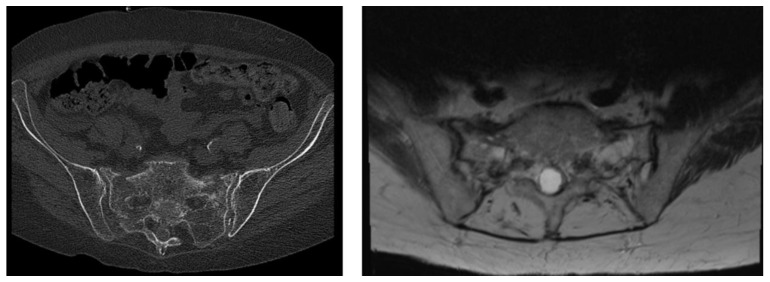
An 82-year-old patient presenting with a fragility fractures of the pelvis (FFP) type IV or OF4 (OF-Pelvis classification) with displaced bilateral posterior lesions. The bilateral fractures are seen in the computer tomography (CT) (left side) and magnetic resonance imaging (MRI) (right side). The patient suffered from a low-energy trauma, falling on her back.
